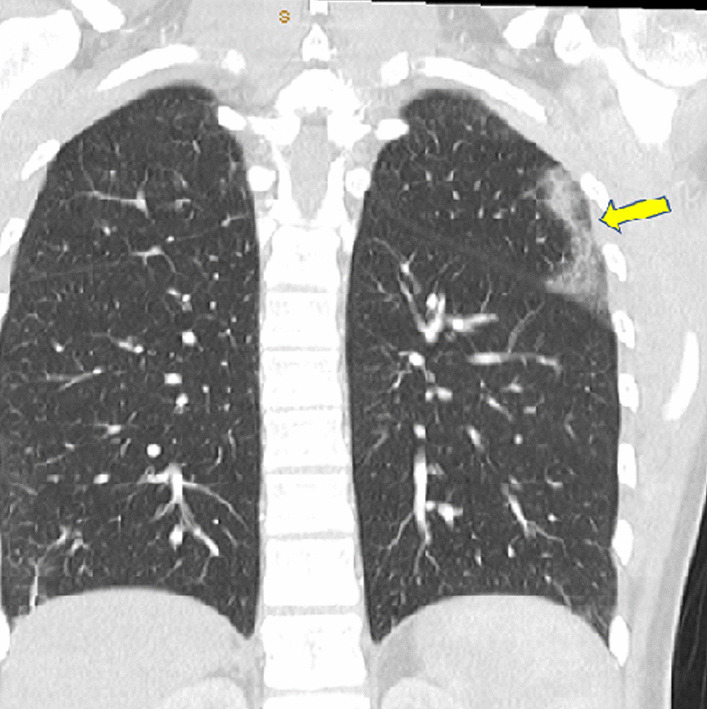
Case 1. Chest computed tomography (CT) coronal view. Nonspecific sub pleural pulmonary infiltrates in the left upper lobe (yellow arrow). CT guided needle biopsy of this lesion revealed eosinophilic infiltrates consistent with acute eosinophilic pneumonia
